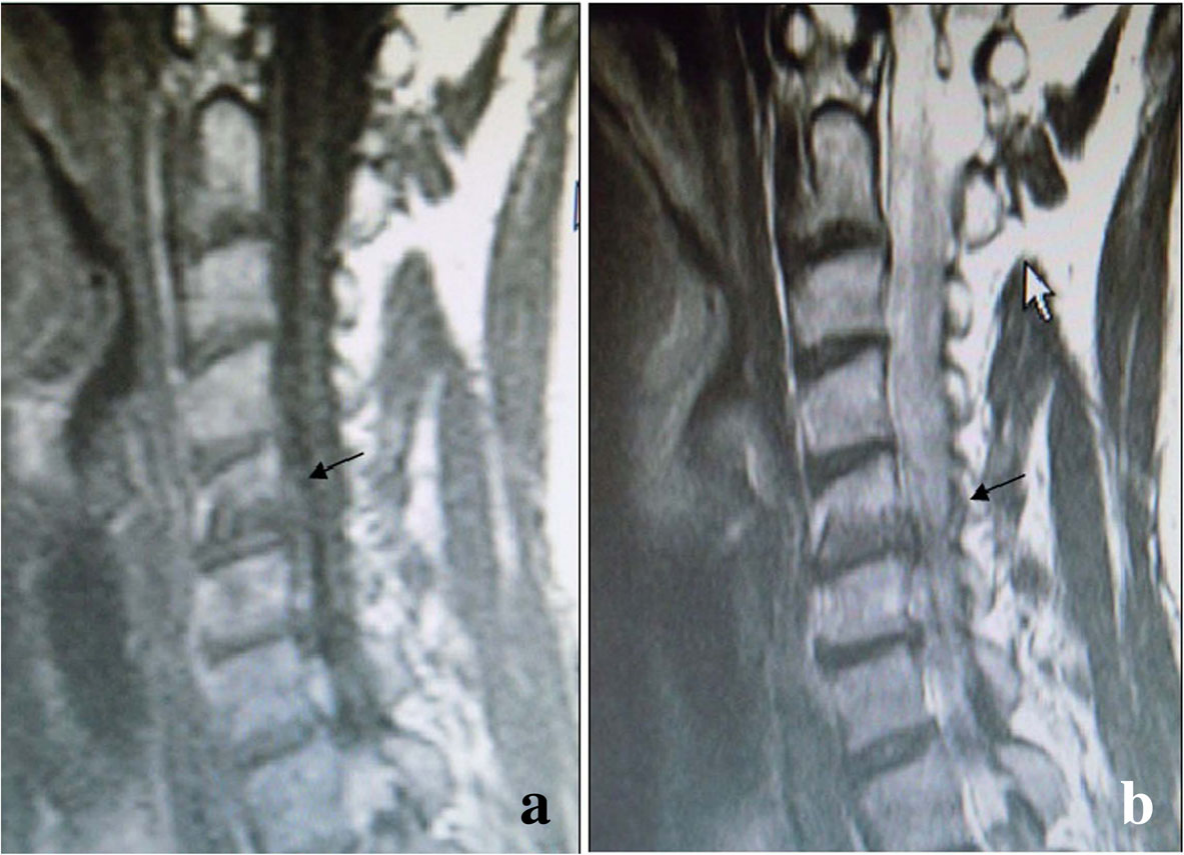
Modic type III changes. Hemispheric low signal intensity on T1-weighted (a) and T2-weighted (b) images is noted on the upper endplate at the C5–6 level in the cervical spine
